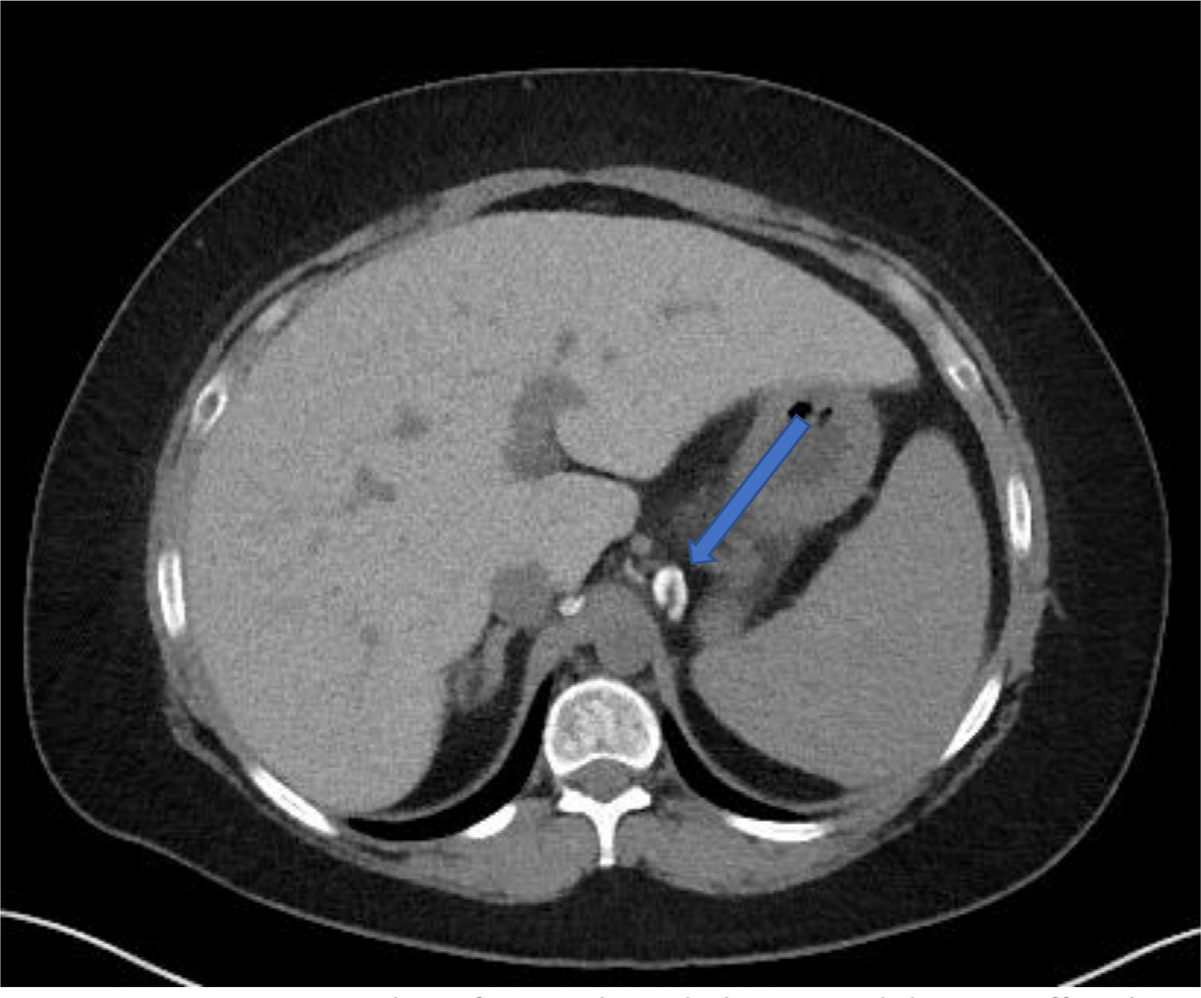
Noncontrast axial CT of image through the upper abdomen. Diffuse hyperattenuation of the liver (HU 88) compared to the spleen (HU 52). Additionally, there are high density upper abdominal lymph nodes (blue arrow). In secondary hemochromatosis, iron deposits in the reticuloendothelial system including the liver, spleen, bone marrow, and lymph nodes resulting in higher attenuation. Interesting in this case, the disease process spared the spleen which is on the upper limits of normal (40-60 HU).
